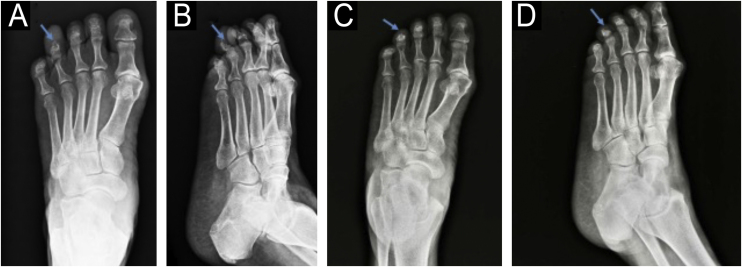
Anteroposterior (A) and lateral (B) radiographs of the left foot before the surgery: increased soft tissue density and bone erosion of the distal phalanx (blue arrow), resembling a “cup” appearance (B). Anteroposterior (C) and lateral (D) radiographs of the left foot two months after the surgery: partial recovery of bone structure.
